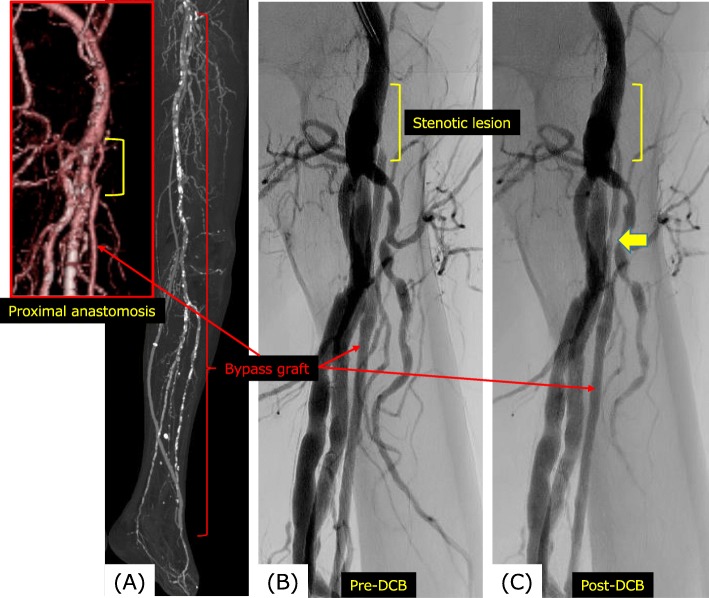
a Computed tomography angiography after distal bypass surgery. The reversed saphenous vein bypass is shown with a red bracket. The proximal anastomotic site was focused in another window. b Intra-arterial digital angiography before percutaneous transluminal arterioplasty with drug-coated balloons (DCBs). The stenosed area of the reversed saphenous vein graft is shown with a yellow bracket and the bypass graft is shown with a red arrow. c Intra-arterial digital angiography after PTA with DCB. The stenotic lesion was successfully dilated using a DCB. Another re-stenotic lesion was shown below without PSV increase, for which we dilated with DCB
